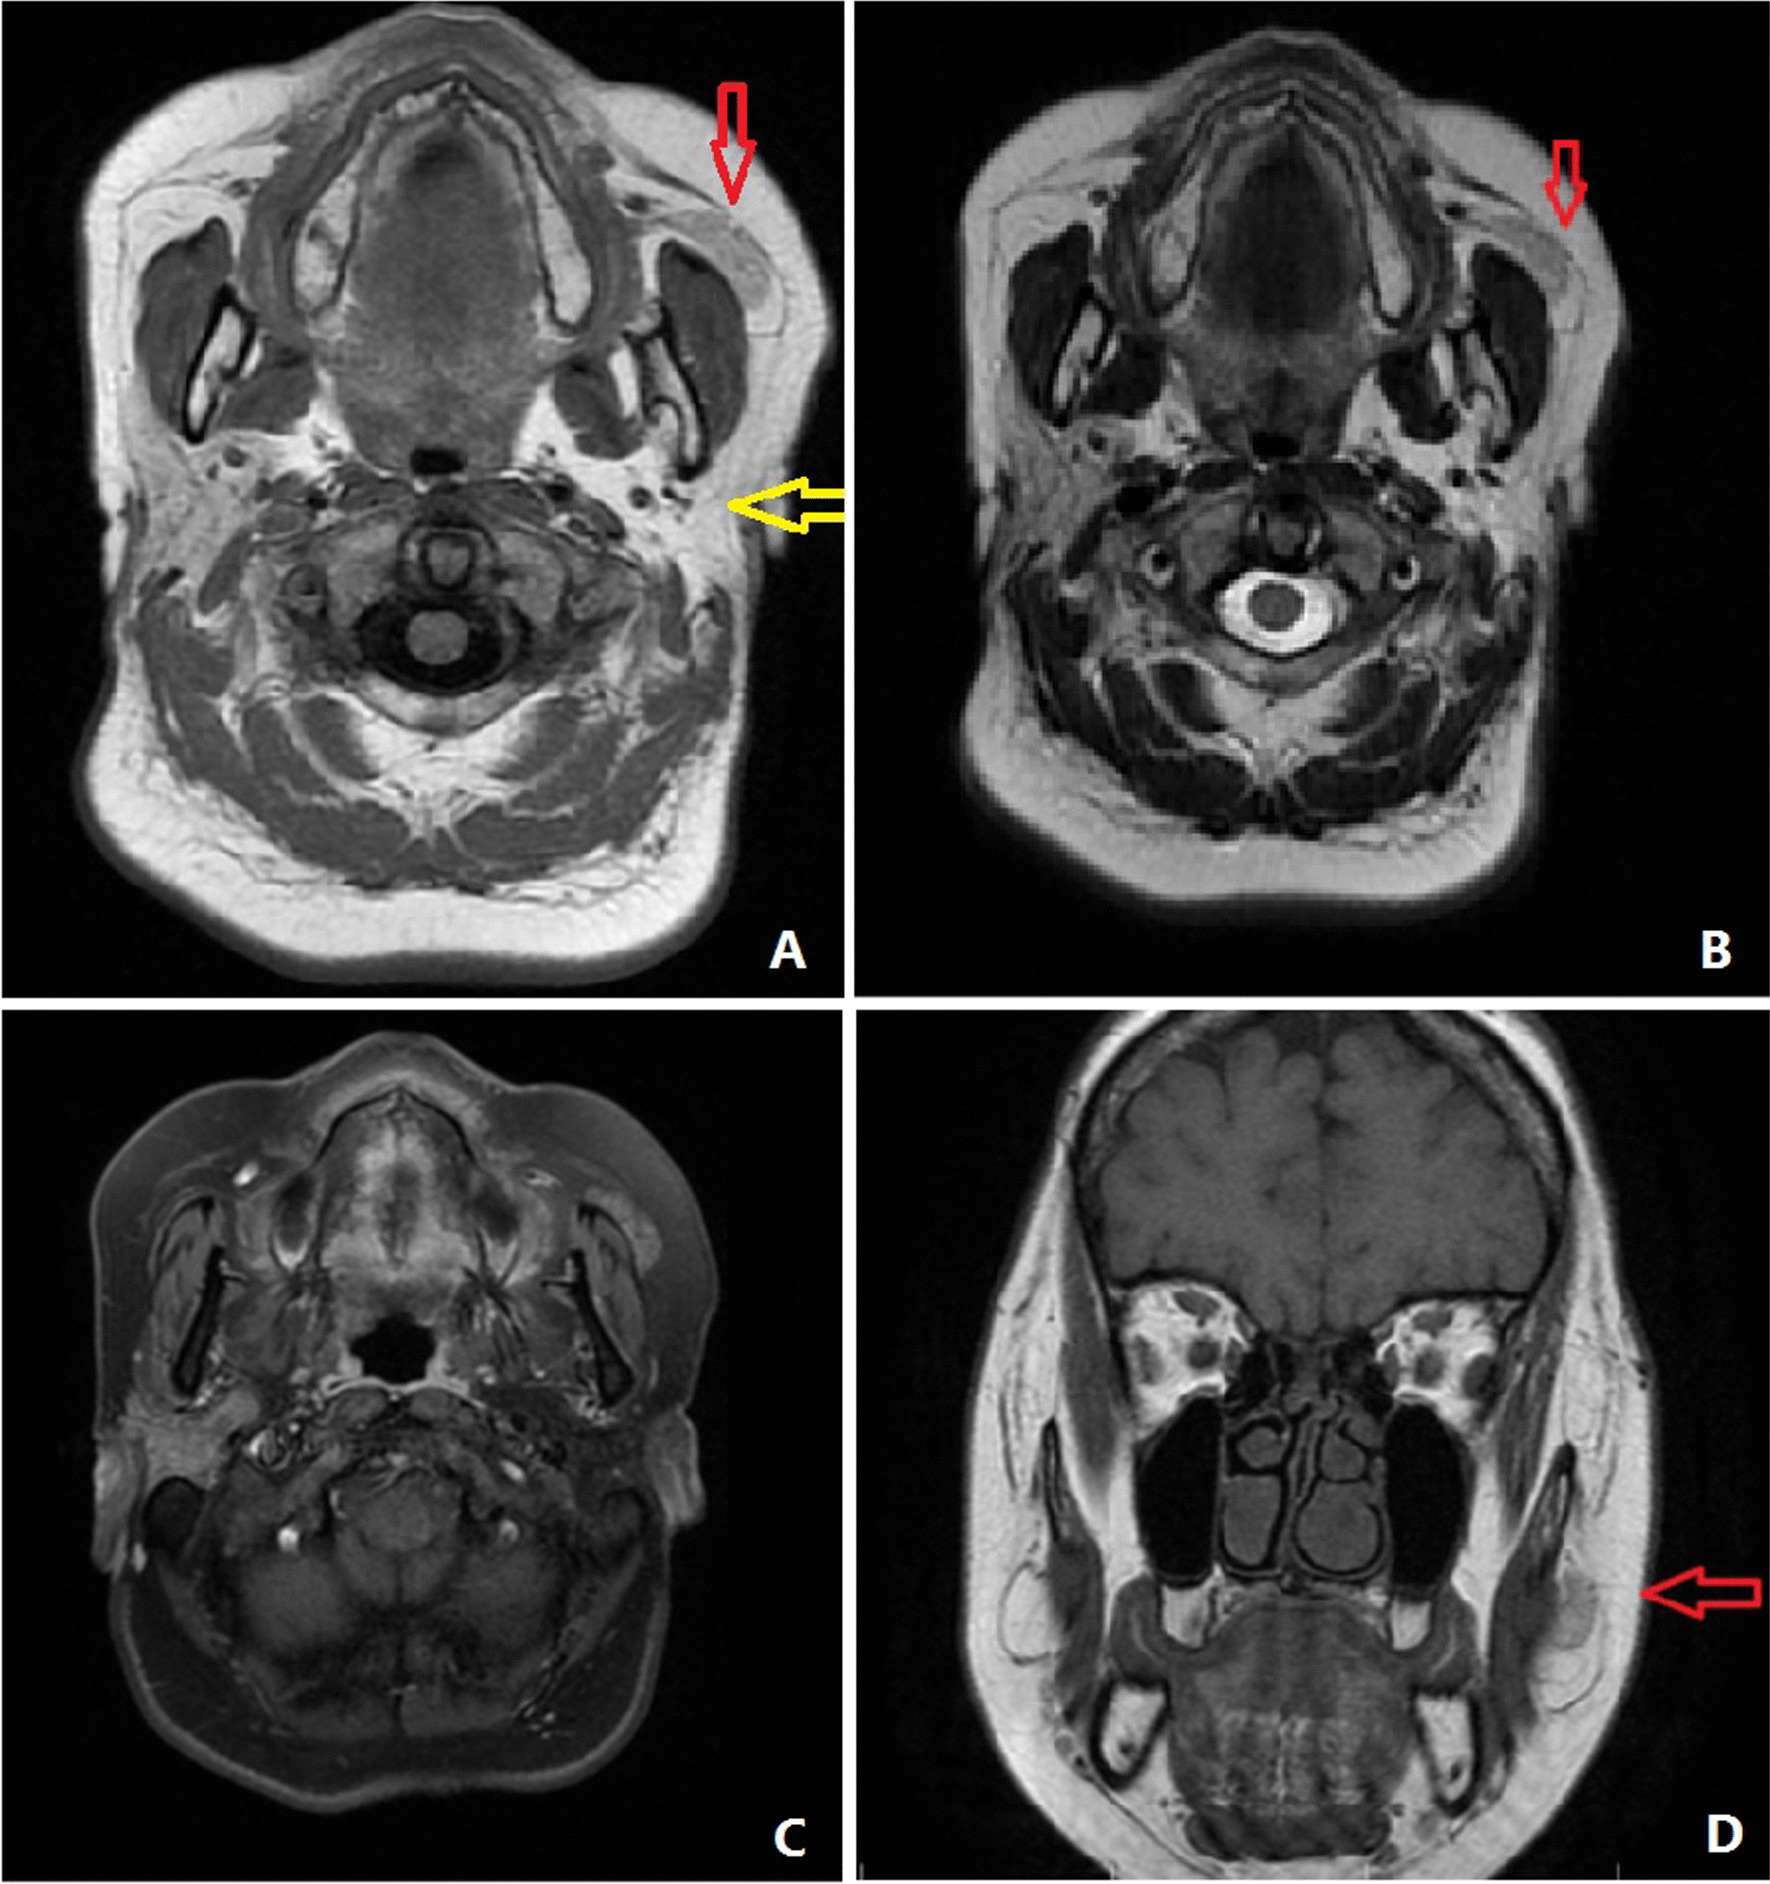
A Axial T1 fluid attenuated inversion recovery (FLAIR) sequence MRI. The left parotid gland is not seen in the normal anatomic area (yellow arrow) and located in left buccal fat (red arrow). B Axial T2-weighted MRI. The left parotid gland is not seen in the normal anatomic area and is located in left buccal fat (red arrow). C Axial T1 fat-saturated with gadolinium. The same mass with enhancement similar to the right parotid gland. D Coronal T1-weighted image, with the mass in left buccal fat (red arrow)
